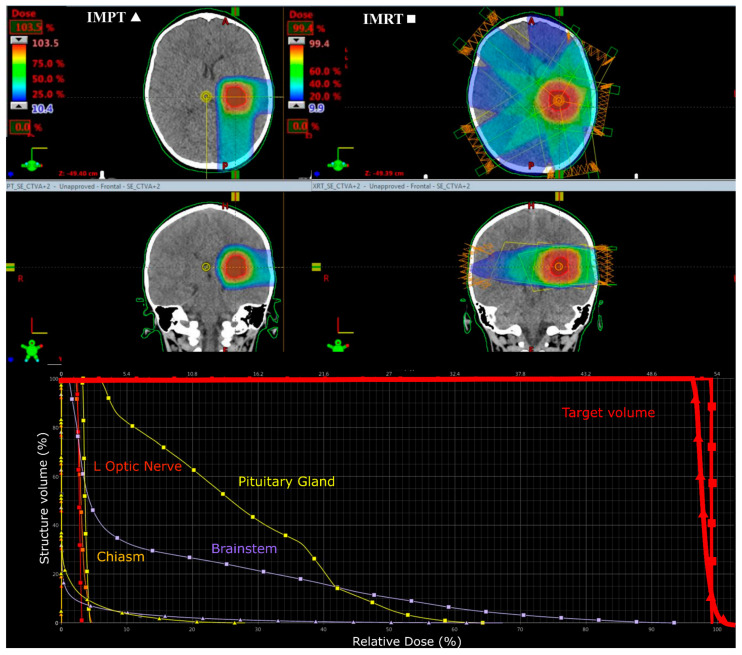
Colourwash dose distributions and dose–volume histograms for a 5-year-old male with supratentorial ependymoma. Typical planning beam arrangements and dose–volume histograms viewed on axial and coronal CT images for intensity-modulated proton therapy (IMPT) (left ▲) and intensity-modulated photon radiotherapy (IMRT) (right ■) plans.
